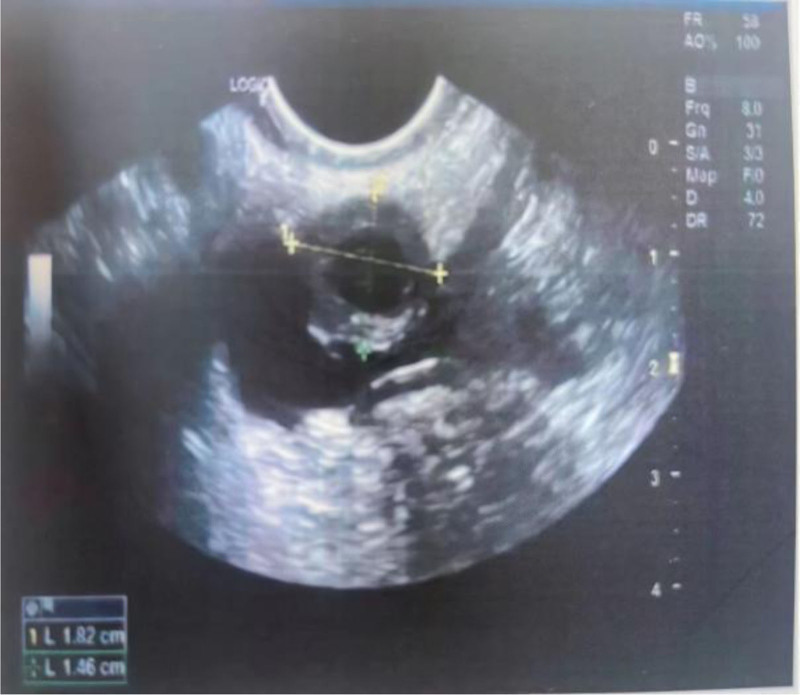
Transvaginal ultrasound scan showing a mixed-echo mass was in the middle of the pelvic cavity, measuring 18 × 22 mm.
